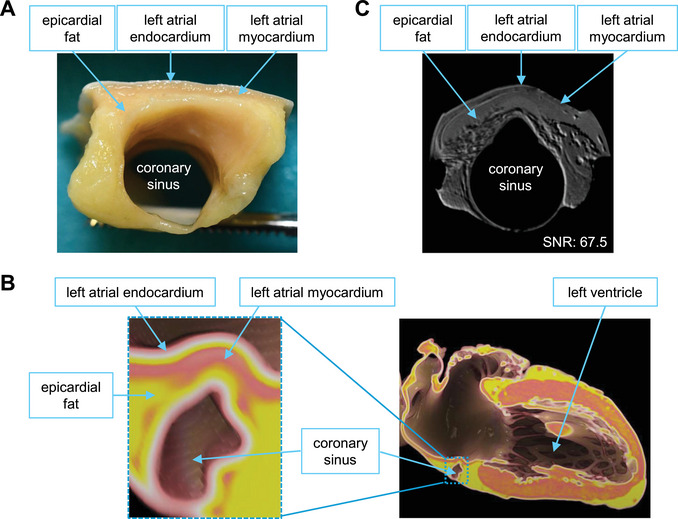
MRI image of an *ex vivo* human heart tissue. (A) Photograph of the human heart tissue. (B) Location of the tissue within the human heart. (C) MRI image of the human heart tissue embedded in Fluorinert. Detailed anatomical structures, including the epicardial fat, the left atrial endocardium, and the left atrial myocardium, can be visualized in the MRI image (SNR: 67.5). The scan time was 11.5 hr.
